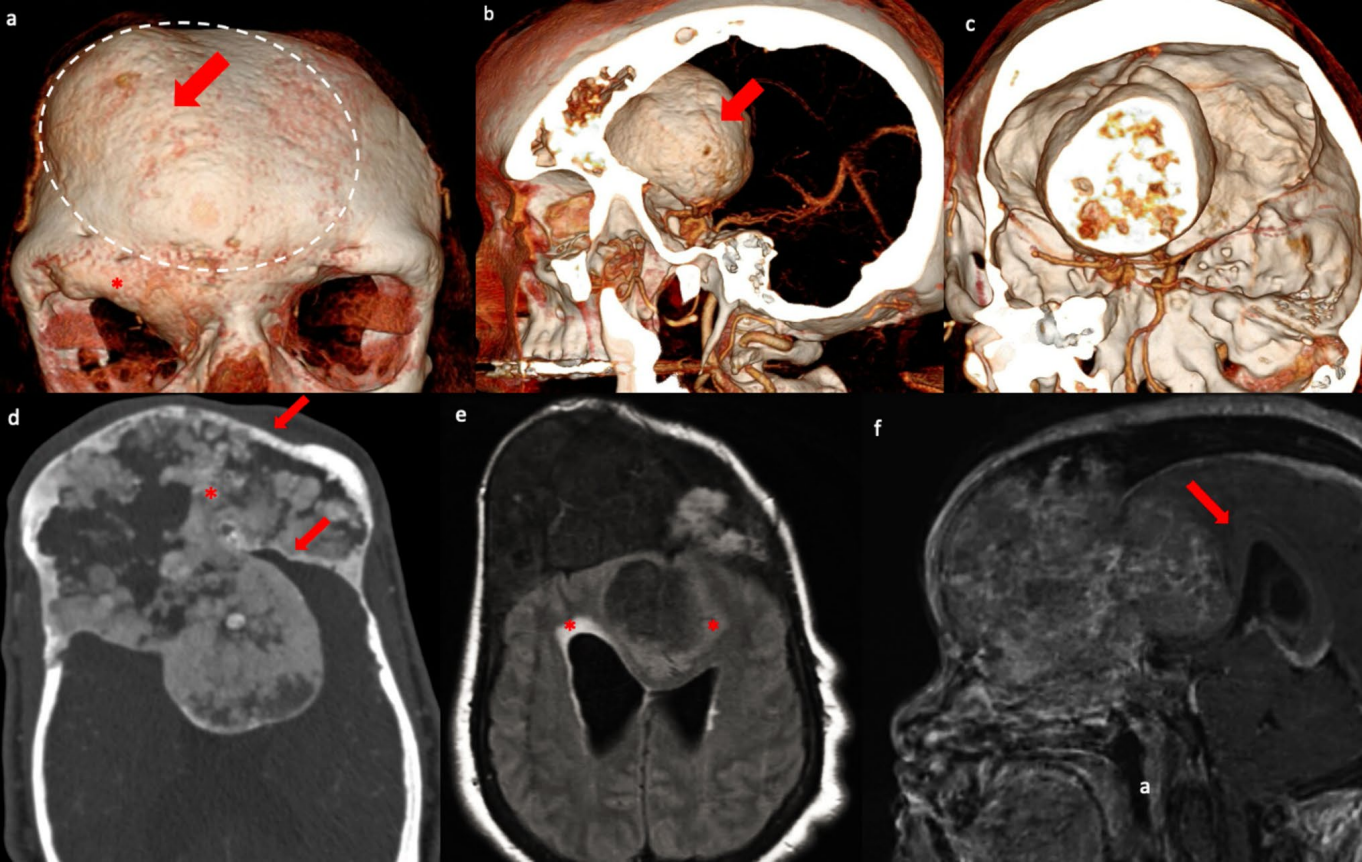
Preoperative imaging work-up: fine-cut computed tomography (CT), CT angiography (CTA) and brain magnetic resonance image (MRI). a Coronal 3D-reconstruction from CT scan demonstrated a large bony deformity in the right portion of the anterior calvarium (arrow) and the right superior orbital rim (asterisk). The white dashed circle highlights the planned bifrontal craniectomy. b Sagittal 3D-reconstruction from CTA showed a large intracranial pedunculated mass (arrow) within the anterior and middle cranial fossae. c Coronal 3D-reconstruction from CTA revealed the anatomical relationship between the intracranial mass and the major cerebral arteries. CTA did not identify any significant arterial feeders to embolize prior to surgery. d Axial CT scan with bone window settings revealed complete obliteration of the frontal sinus due to the bony growth (asterisk), along with disease extension into its anterior and posterior wall (arrows). e Axial T2-FLAIR MRI highlighted hyperintense signals in the periventricular area and around the intracranial lesion (asterisks). The lesion’s size had remained stable in comparison with the previous imaging; however, the FLAIR signal change represented a new finding. f Sagittal T1-postcontrast MRI demonstrated an extensive, irregularly enhancing lesion involving the frontal, sphenoidal, and ethmoidal regions, causing severe cerebral mass effect, particularly on the corpus callosum (arrow)
